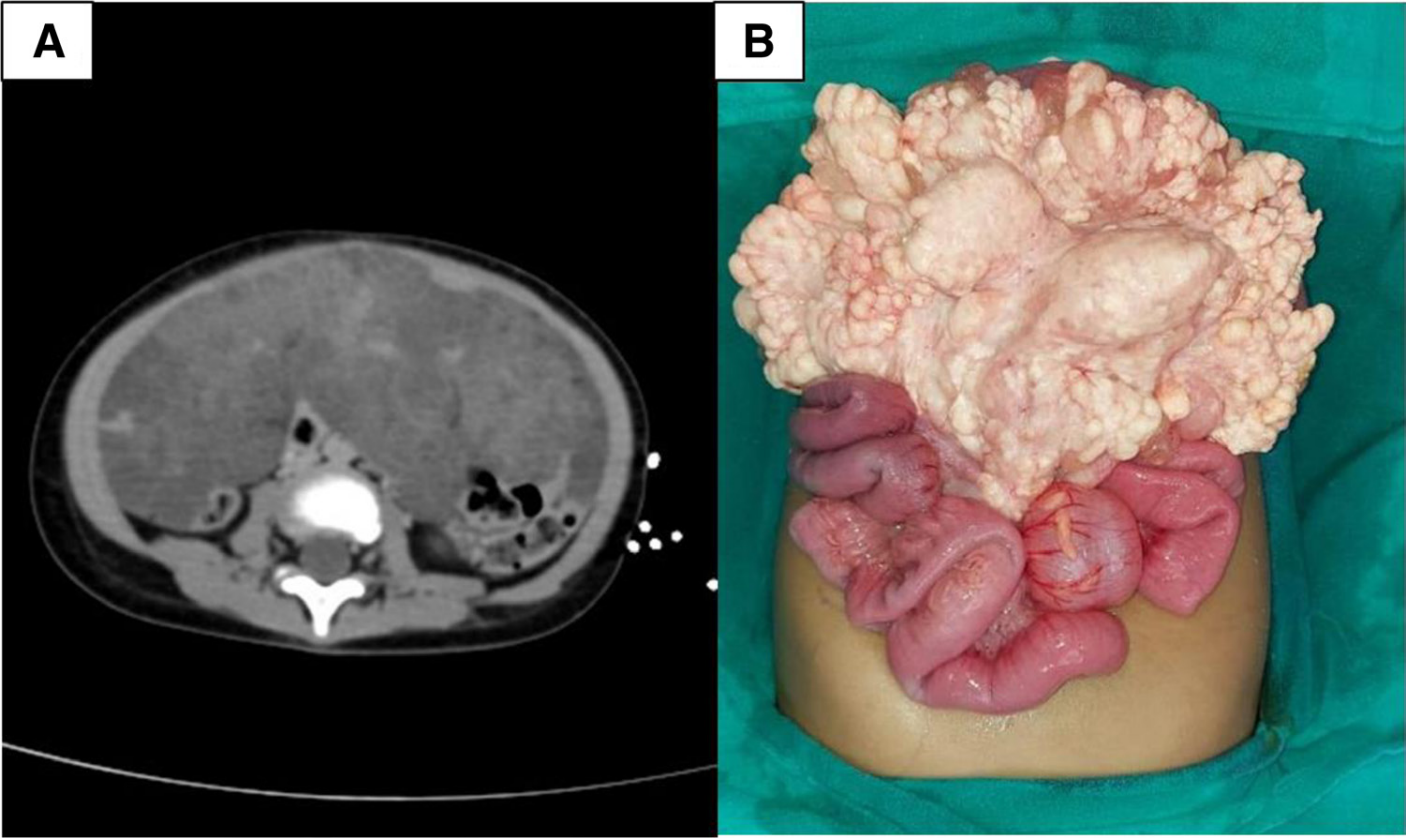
Positron emission tomography-computed tomography scan image and intraoperative image. A) Positron emission tomography-computed tomography scan image showing large ill-defined mass measuring 16 × 13.2 × 6.7 cm in size seen in relation to the abdominal mesentery and involving umbilical, infra umbilical, and hypogastric region with extension into the bilateral ileal fossa. B) Intraoperative image of multiloculated cyst of variable size arising from mesentery.
